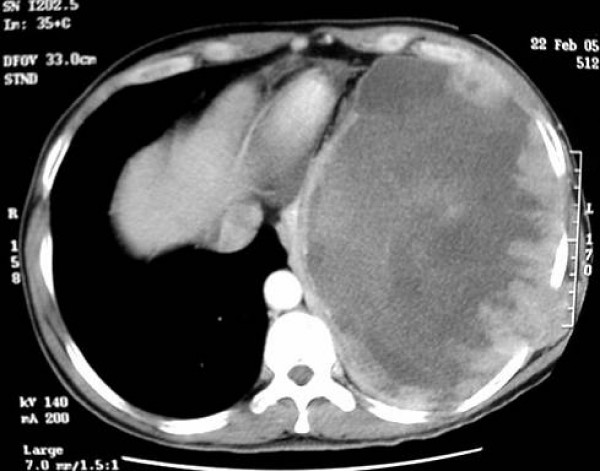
Computed tomography scan of the chest showing a large synovial cell sarcoma with extensive involvement of surrounding structures.
